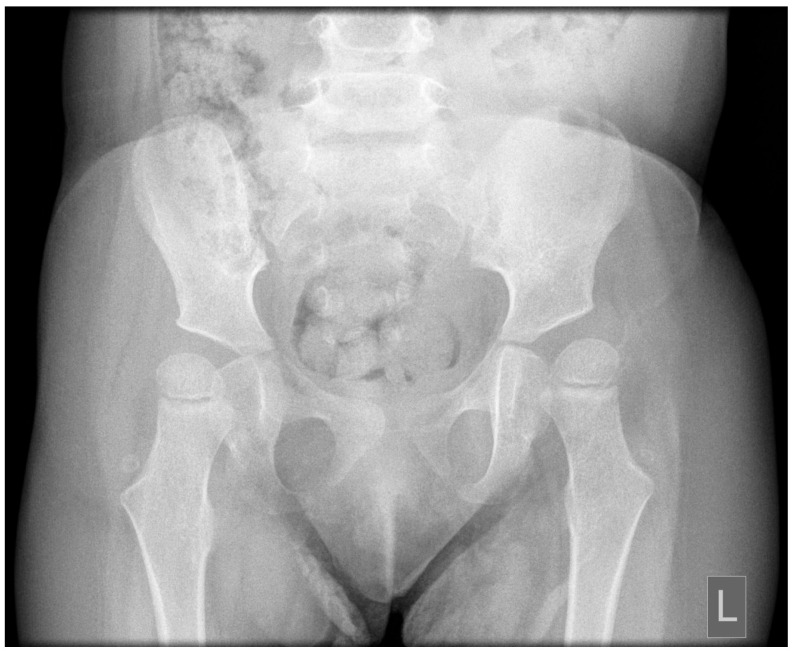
Pelvic radiograph demonstrating asymmetric lateral migration of the femoral heads, more pronounced on the left side, consistent with early hip displacement.
